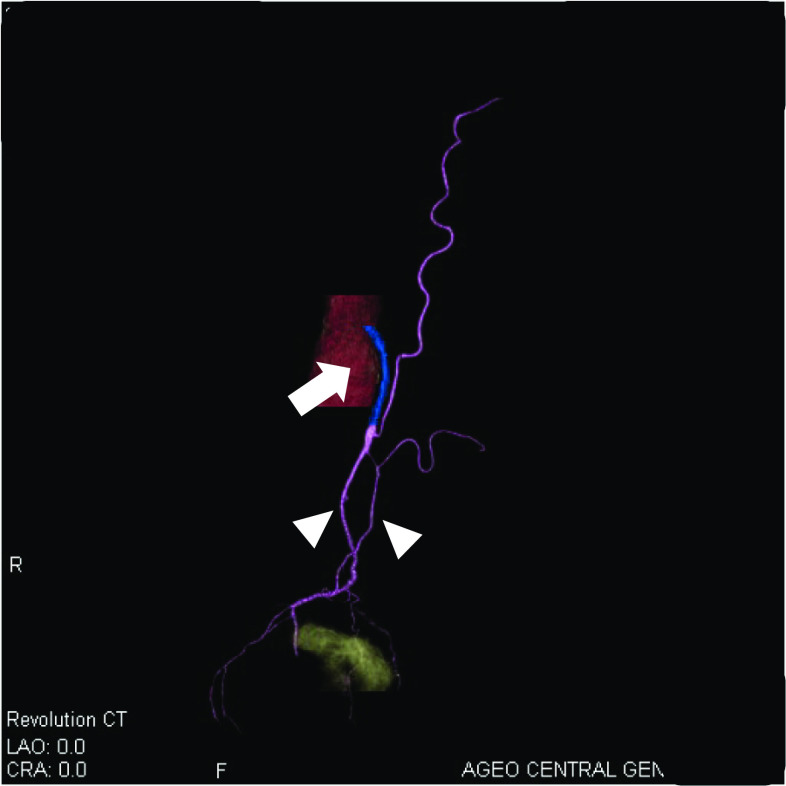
CT angiography revealed occlusion of the aorta just below the origin of the inferior mesenteric artery (white arrow) and the dominant vessels of the tumor were identified as SRA and S2 (white arrowhead)
